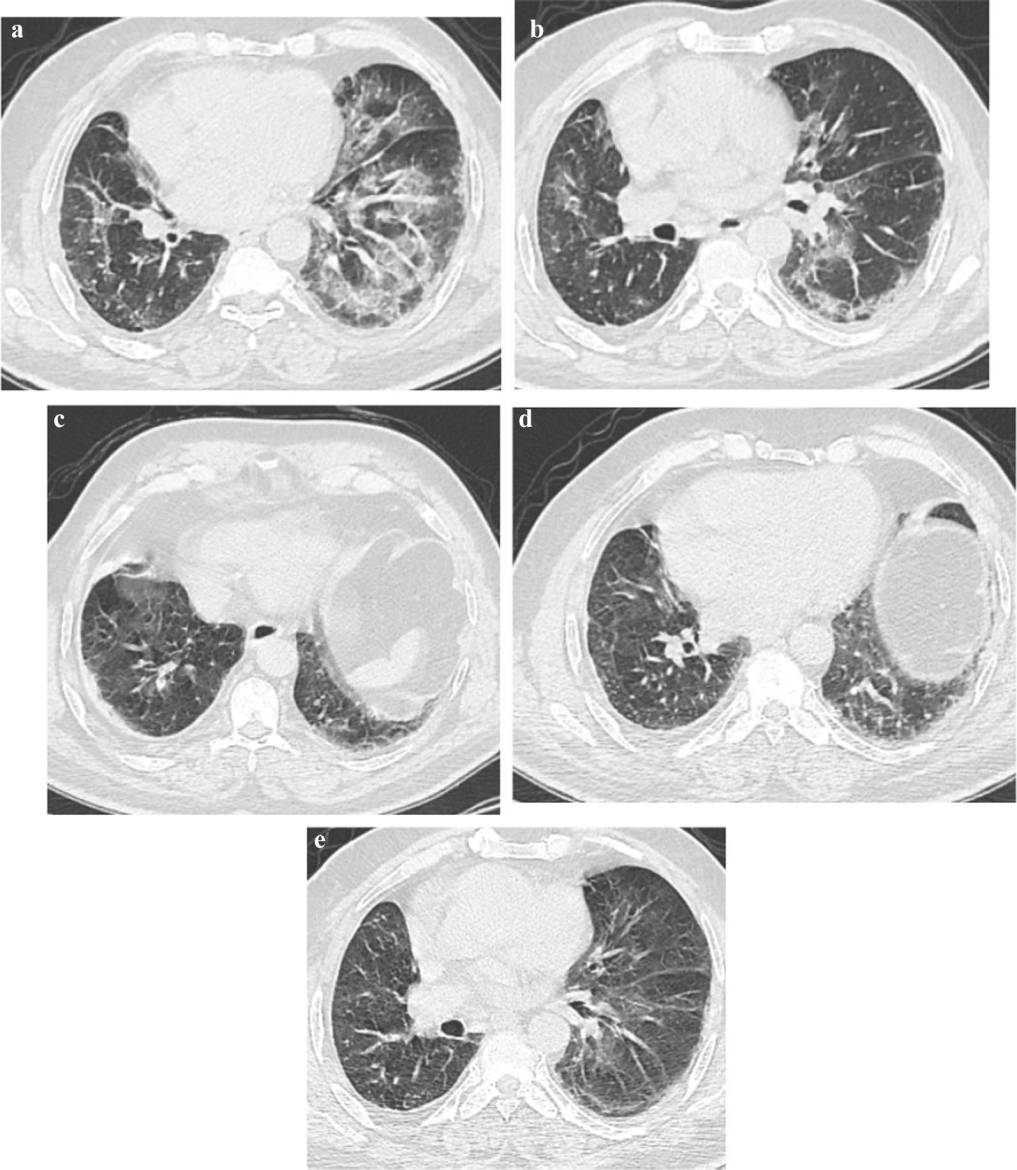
A 65-year-old female known IHD presented with anosmia, loss of taste, cough, fever, respiratory distress, diarrhea and myalgia at May 2020 and positive PCR for COVID 19 infection, a and b noncontrast CT axial cuts show bilateral faint ground glass opacities with crazy paving sign. The patient diagnosed as COVID 19 infection with CT score severity: 20. Second infection dated May 2021 the patient presented with fever, diarrhea and myalgia with positive PCR test for COVID 19 infection. c–e noncontrast CT chest images show bilateral patchy subpleural faint ground glass opacities and reticulations. The patient diagnosed as COVID 19 re-infection with CT severity score: 5
